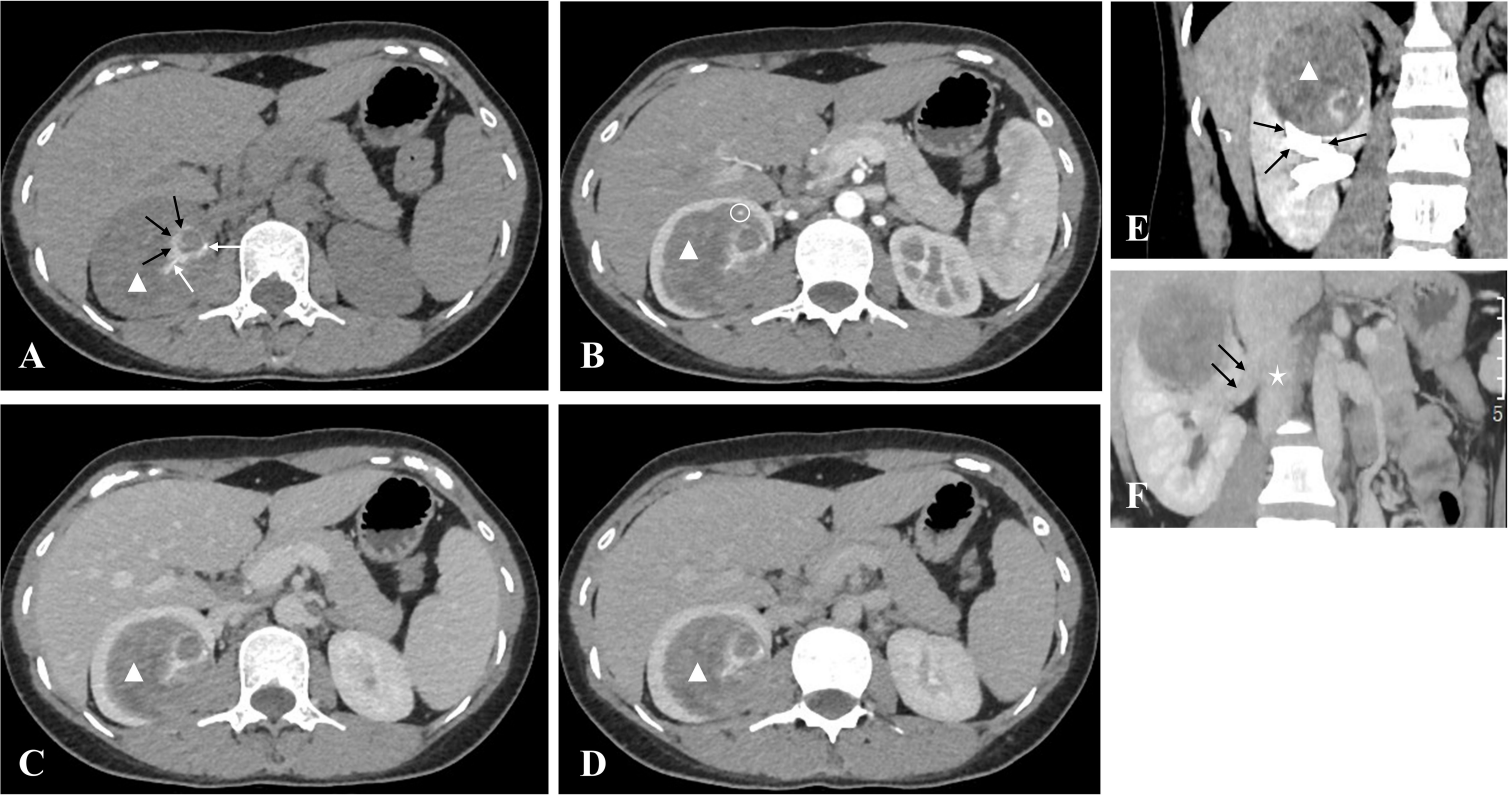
Non-contrast and contrast-enhanced abdominal computed tomography (CT) images of the right renal anaplastic lymphoma kinase-rearranged renal cell carcinoma. (A) Axial non-contrast CT shows a round right renal mass (white triangle) located in the medulla, measuring 54×55×65 mm with heterogeneous hypodensity (mean 46 HU). Multiple punctate and patchy calcifications (white arrow) and flocculent slightly hyperdense areas (black arrow) are visible within the mass. (B) Axial corticomedullary-phase CT demonstrates mild heterogeneous enhancement of the mass (mean 52 HU; enhancement ratio 1.13 vs. non-contrast, white triangle), with a feeding artery branch (white circle) originating from the right renal artery. (C) Axial nephrographic-phase CT shows increased enhancement of the mass (mean 58 HU; enhancement ratio 1.26 vs. non-contrast, white triangle). (D) Axial excretory-phase CT shows further mild enhancement of the mass (mean 61 HU; enhancement ratio 1.33 vs. non-contrast, white triangle). (E) Coronal excretory-phase CT confirms the medullary location of the mass (white triangle) and its compression of the upper renal calyces (black arrow). (F) Oblique coronal maximum intensity projection image (nephrographic phase) shows no filling defects in the right renal vein (black arrow) or inferior vena cava (white star).
